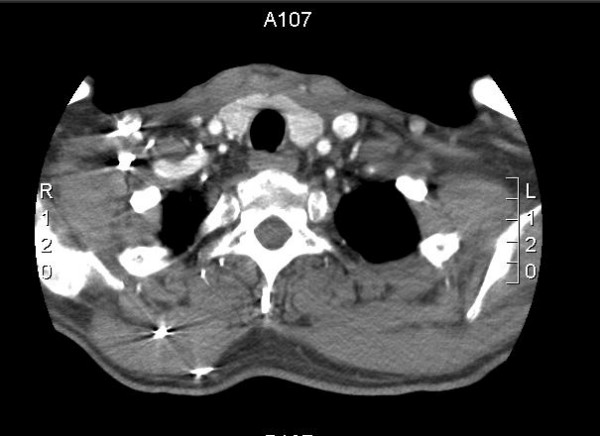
Horizontal CT view of the neck at the level of suprasternal notch (about 6 weeks after surgery). This shows complete resolution of the inflammatory process.
